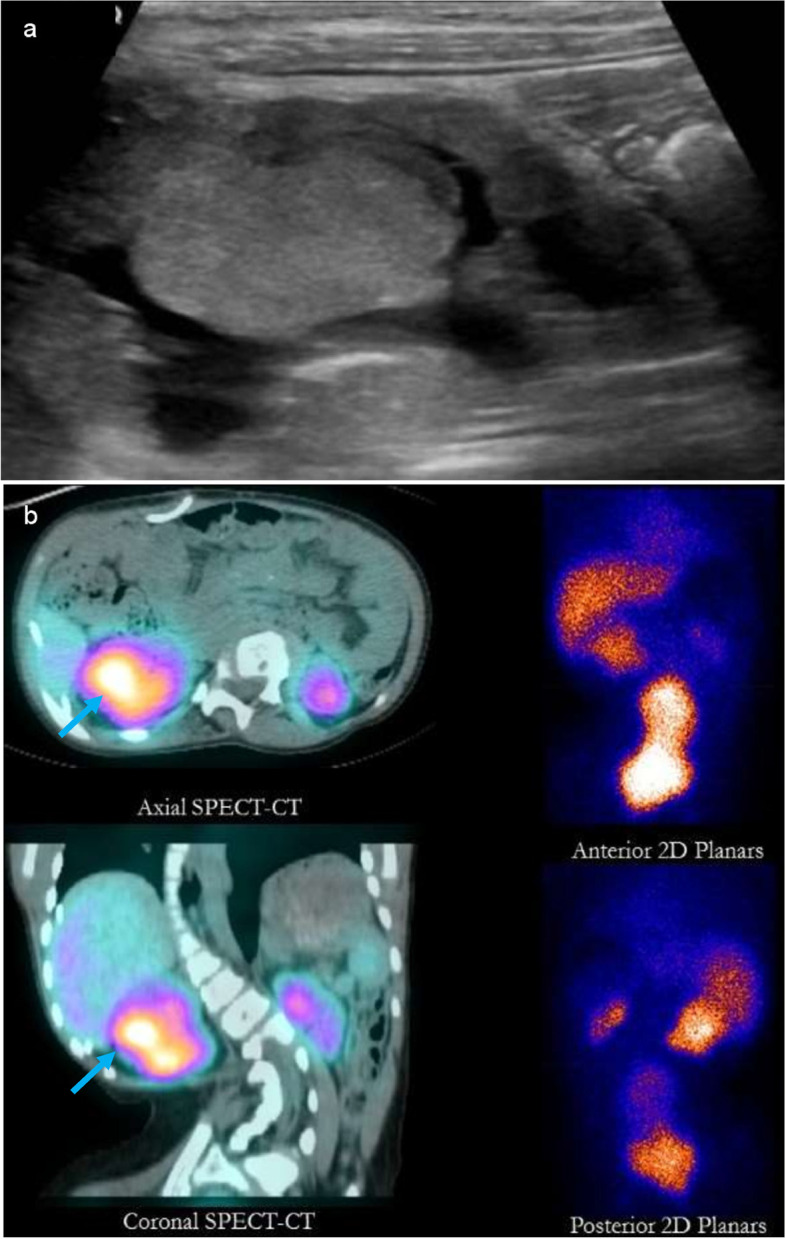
A US images show a large echogenic renal mass on the right. Both kidneys showed loss of cortico-medullary differentiation and cortical defects in keeping with renal scars. B 9-year-old girl with neurogenic bladder and severe bilateral renal scarring and renal masses on US (the largest of which is showed in Fig. 3 (A)). Those masses are showing uptake of DMSA in a pattern consistent with FPT on the right side. Uptake is barely seen on 2D planar imaging. In this case, 3D SPECT-CT (white arrows) offers better localization and improved sensitivity, specificity and reader confidence compared to 2D planar imaging. This is even more evident in this case with severe spinal deformity
